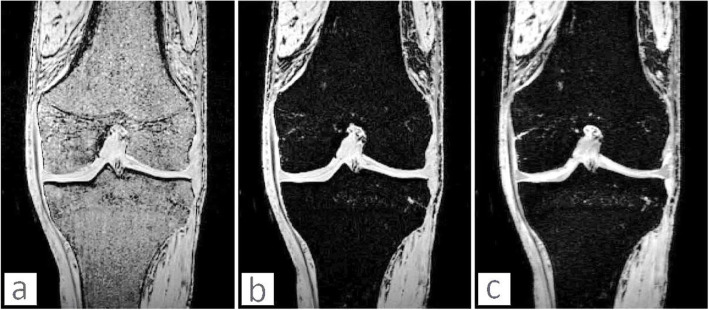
Acquired MRI sequences: a T1 3D, b T1 3D with fat saturation, c T2 3D
